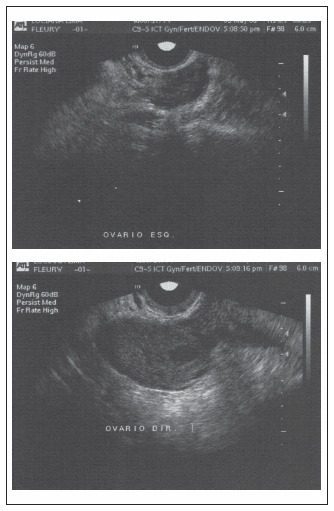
Transvaginal ultrasound on the first intrauterine pregnancy. The right ovary (image on the right) contains a cyst, which is probably the corpus luteum. No cysts are observed in the left ovary (image on the left).
